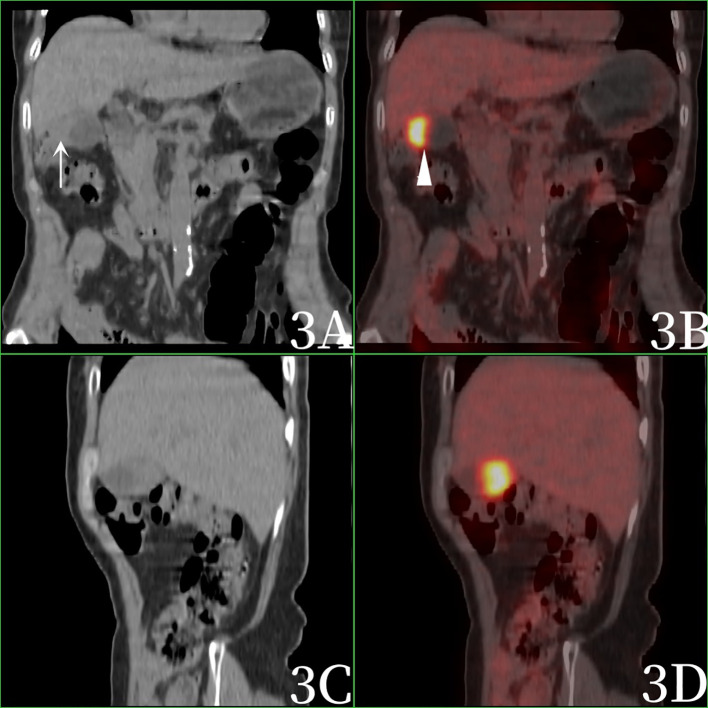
Female, 69 years old. 3A (coronal CT), 3B (coronal fusion), 3C (sagittal CT), and 3D (sagittal fusion). Imaging was performed 1h after 18F-FDG injection. PET/CT showed eccentric and localized thickening of the gallbladder body wall (arrow), nodular soft tissue density shadow, increased radioactive uptake, and SUVmax was 10.2 (arrow).
